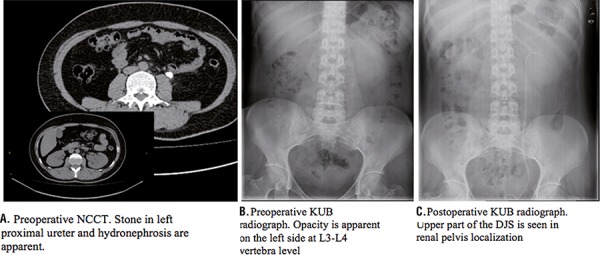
Preoperative KUB radiograph and non-contrast enhanced abdomen CT and postoperative KUB Radiograph.
